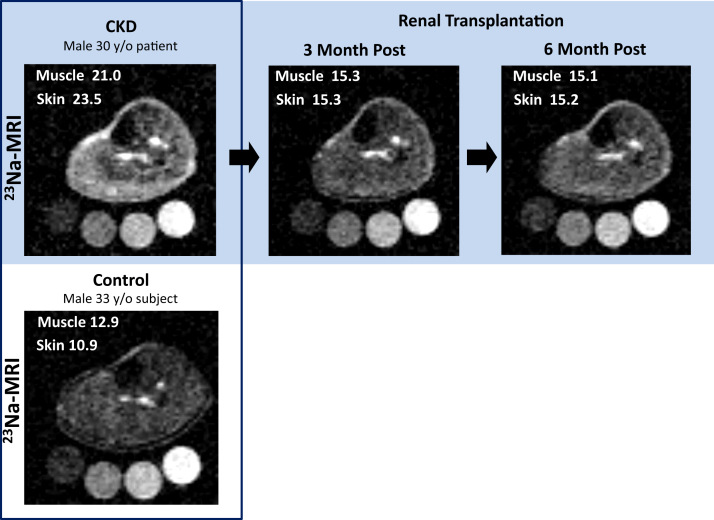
Representative ^23^Na–magnetic resonance images (^23^Na-MRIs) of a chronic kidney disease (CKD) patient (pre– and post–renal transplantation), and its age-matched control person. Upper panel shows ^23^Na-MRI of the left lower leg of a 30-year-old man with CKD stage 5 before (left image) as well as 3 months (middle image) and 6 months (right image) after pre-emptive living-donor kidney transplantation. Calibration tubes with 10, 20, 30, and 40 mmol/l are placed below the left lower leg. The brightness of the Na^+^ resonance signal in muscle and skin tissue is markedly reduced following renal transplantation — reaching signal intensities close to the ^23^Na-MRI of a 33-year-old healthy control man (see boxed images, lower panel).
